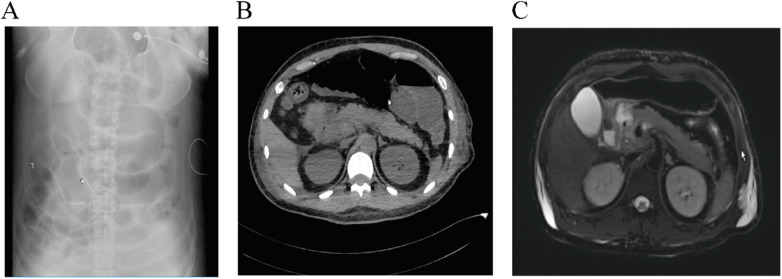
(A) bedside abdominal x-ray reveals small bowel dilatation with gas accumulation and multiple dilated bowel loops; (B) abdominal CT scan shows pancreatic enlargement with blurred surrounding fat planes; (C) abdominal MRI scan demonstrates a full-appearing pancreas with surrounding cotton-wool-like long T2 signals and diffuse restricted diffusion in the parenchyma.
